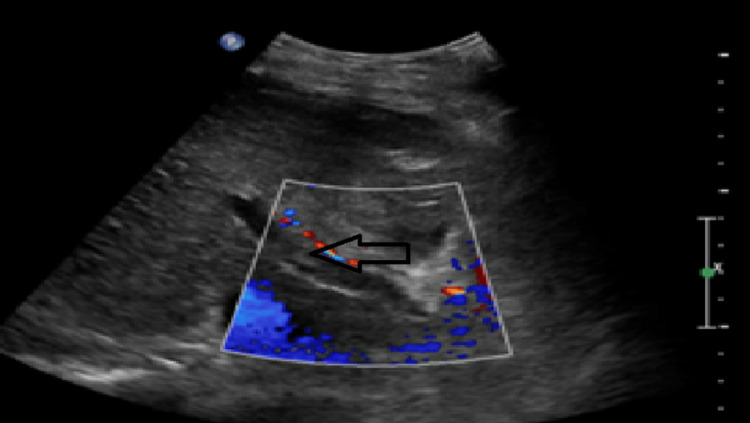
Abdominal ultrasound showing no flow detected in the portal vein suggestive of portal vein thrombosis.
